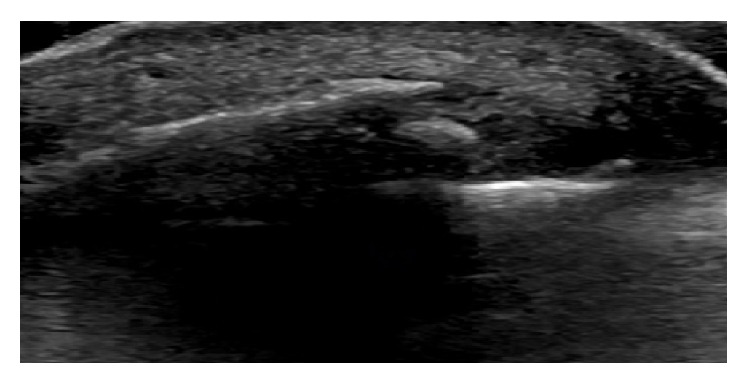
Axial view ultrasound evaluation in a patient with subacute symptoms also shows a large enthesophyte at the insertion of the Achilles tendon.
